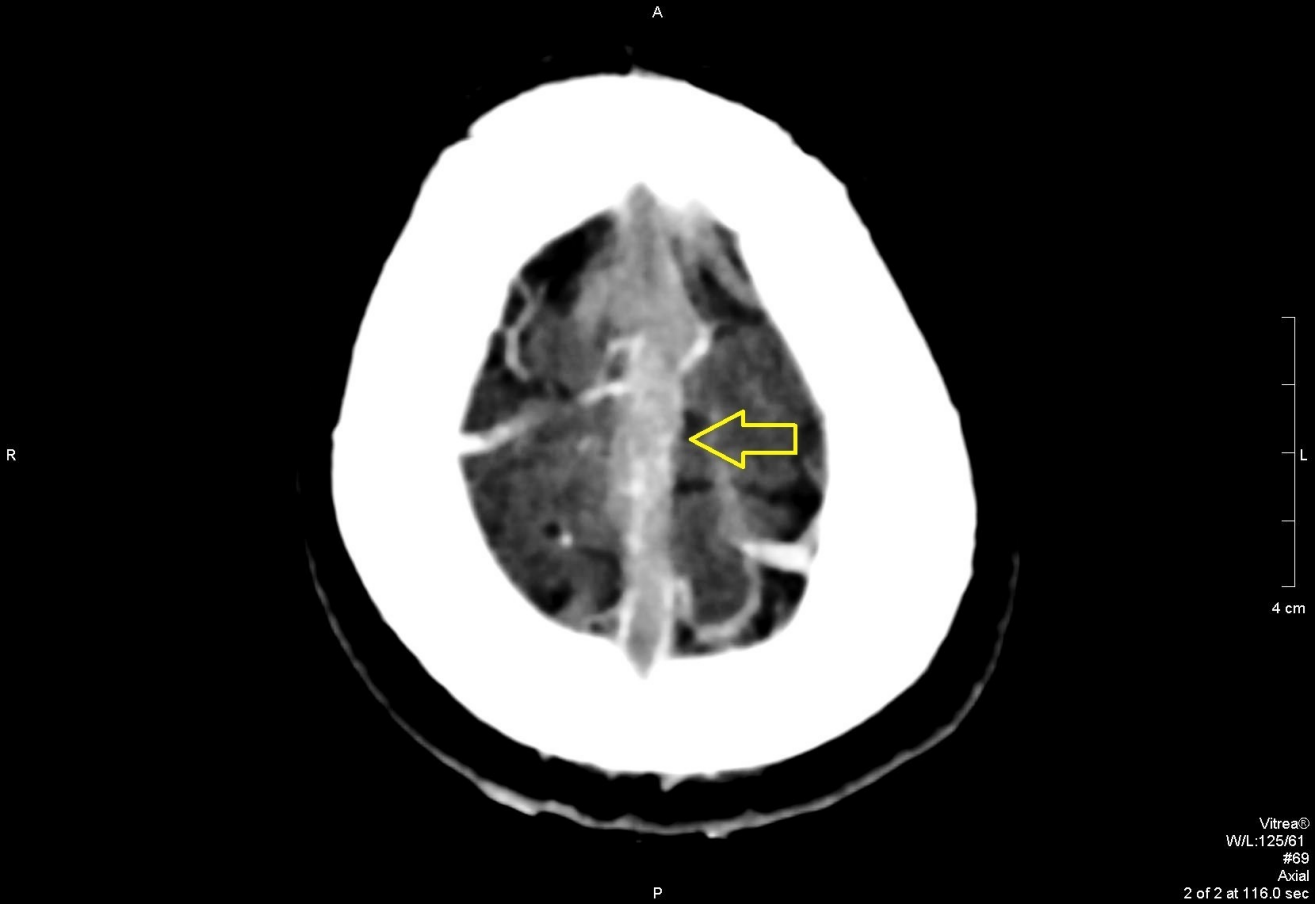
Axial view on contrast CT scan showing superior sagittal sinus blockage CT: computed tomography
